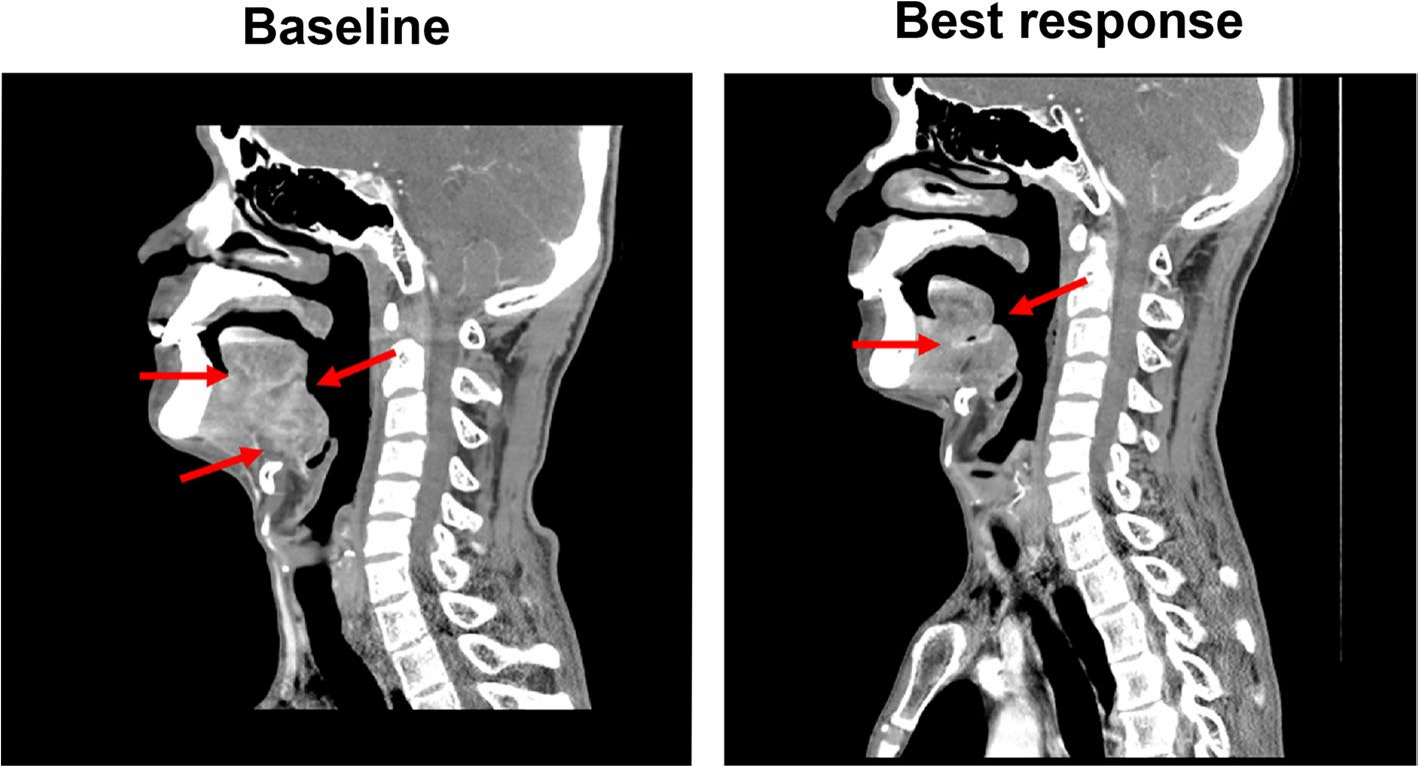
Computed tomography (CT) images of one responder. The patient had primary tongue cancer that was refractory to chemoradiotherapy using triweekly cisplatin 100 mg/m^2^. a Baseline CT at the sagittal plane (b) After 2 months, CT at the sagittal plane revealed a tumor shrinkage. Evaluation at axial planes by RECIST criteria revealed a partial response
